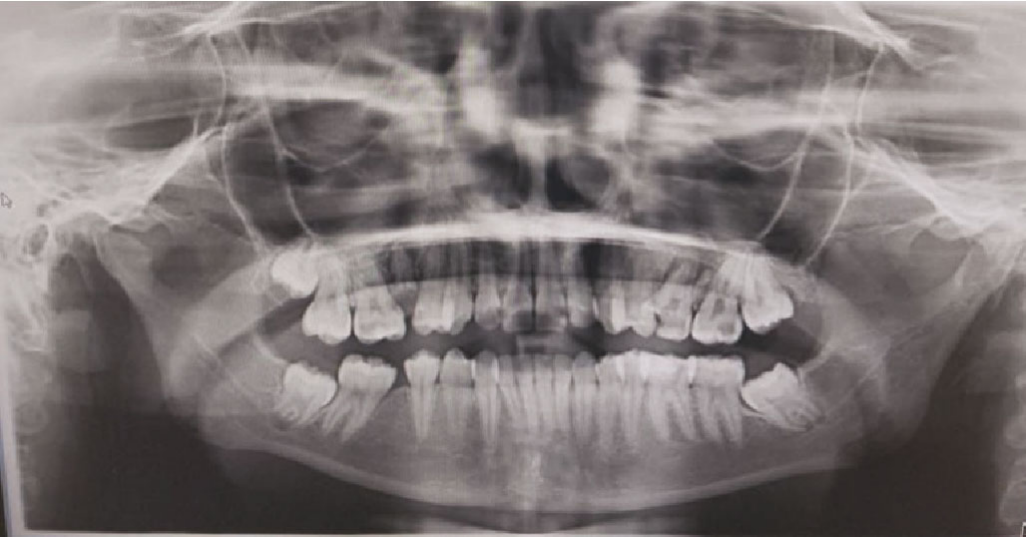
Radiographic assessment of the teeth. Panoramic x-ray of the teeth showing significant bone loss, residual roots, and missing and carious teeth.
